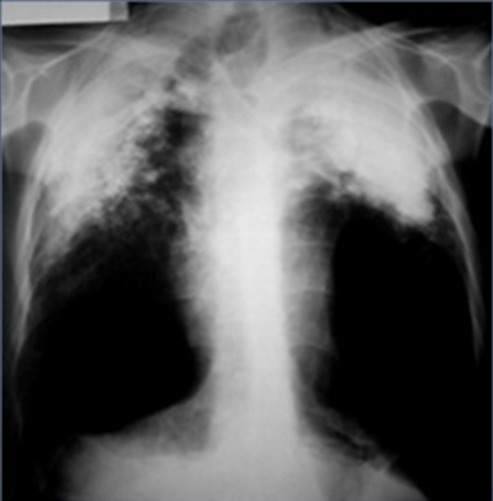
Radiographie thoracique montrant des opacités pseudo-tumorales hétérogènes bilatérales
